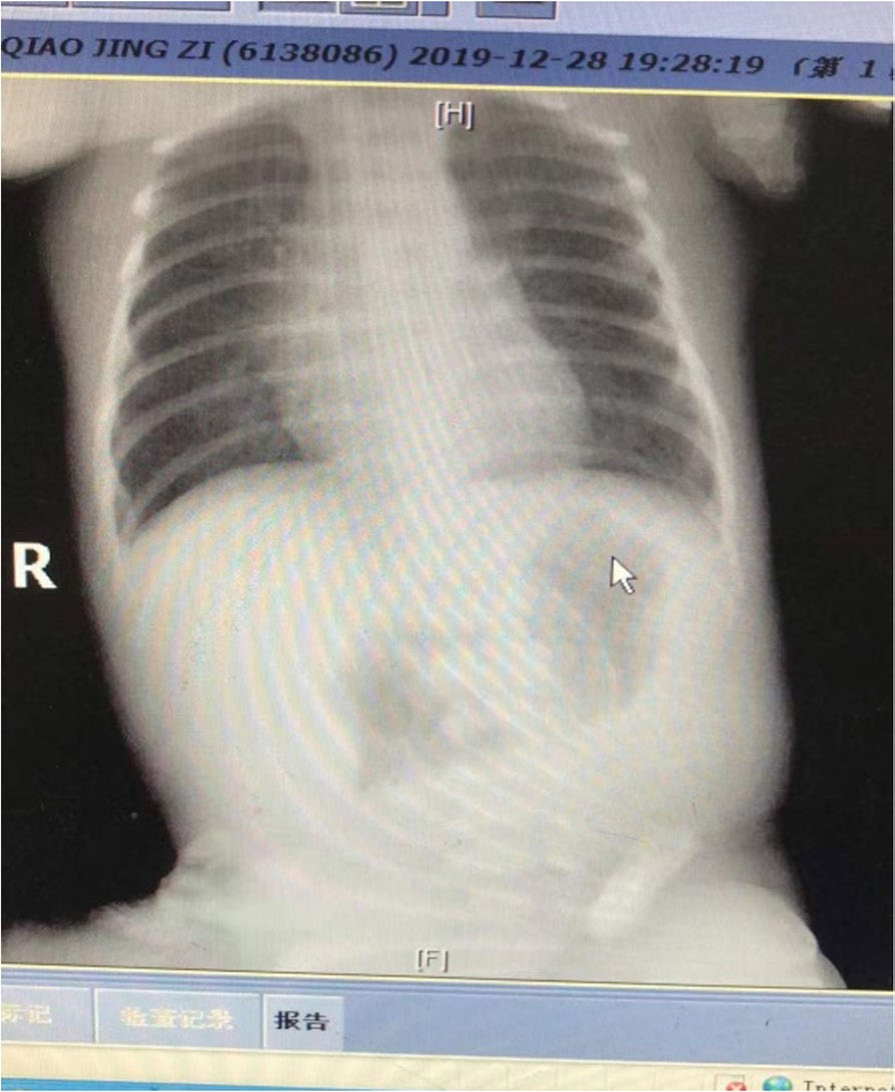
Double bubble sign seen in preoperative X-ray
